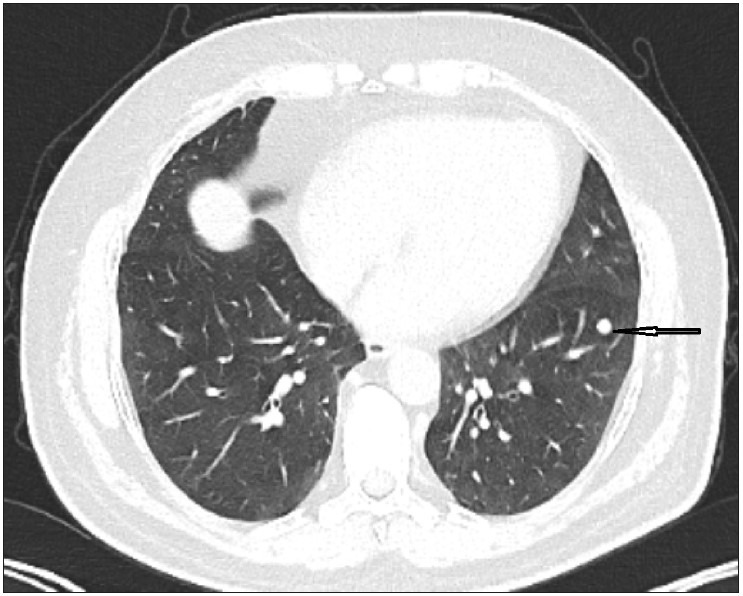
Thorax CT depicting a well-circumscribed lesion 10 cm in diameter in the subpleural area of the left lower lobe (arrow).
